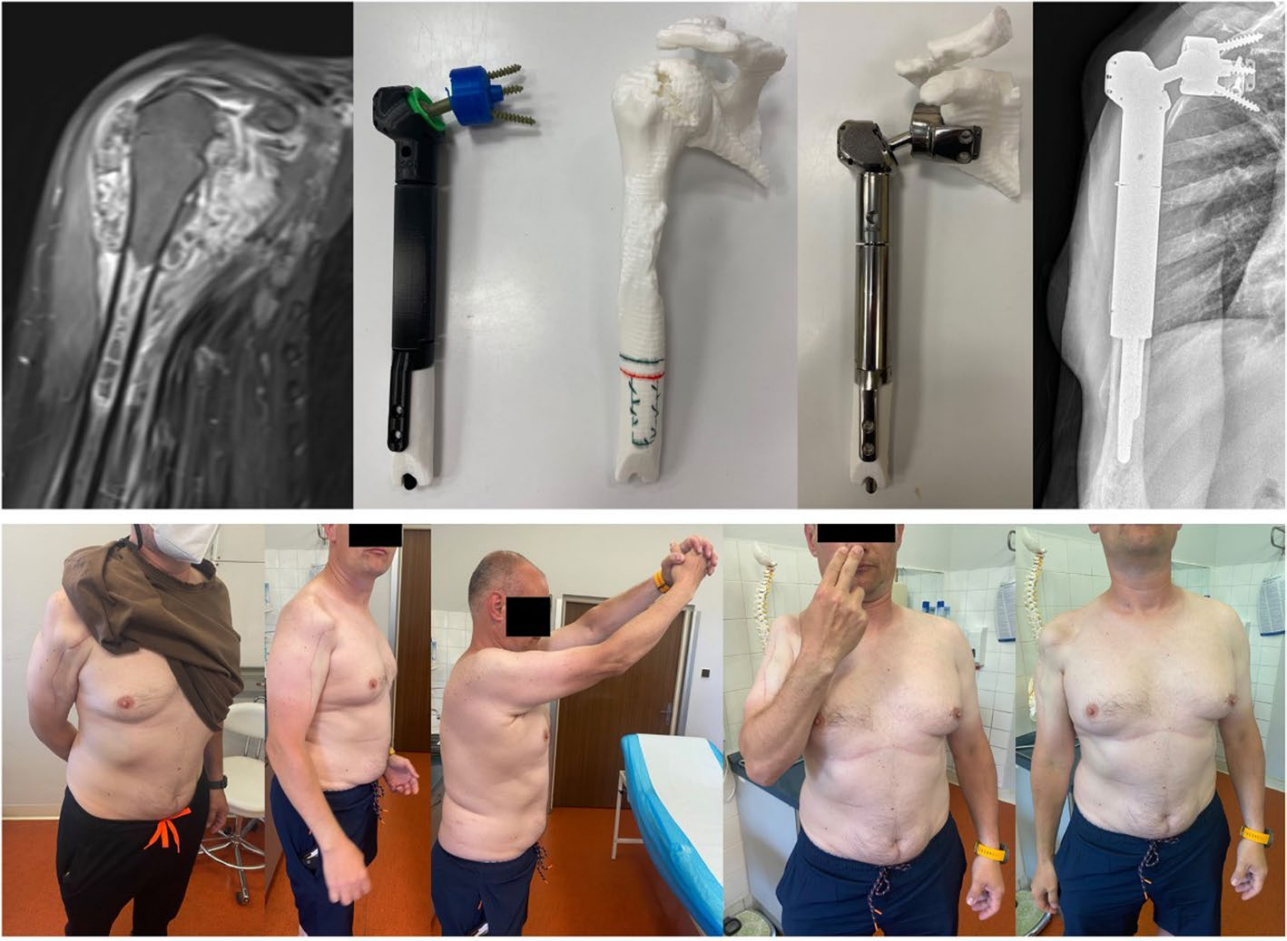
Case example patient 1. Pictures demonstrating the initial tumor presentation on MRI. Dummy and final implant printed. Postoperative radiograph showing good implant positioning and fixation. Clinical images of the patient’s postsurgical right arm function
